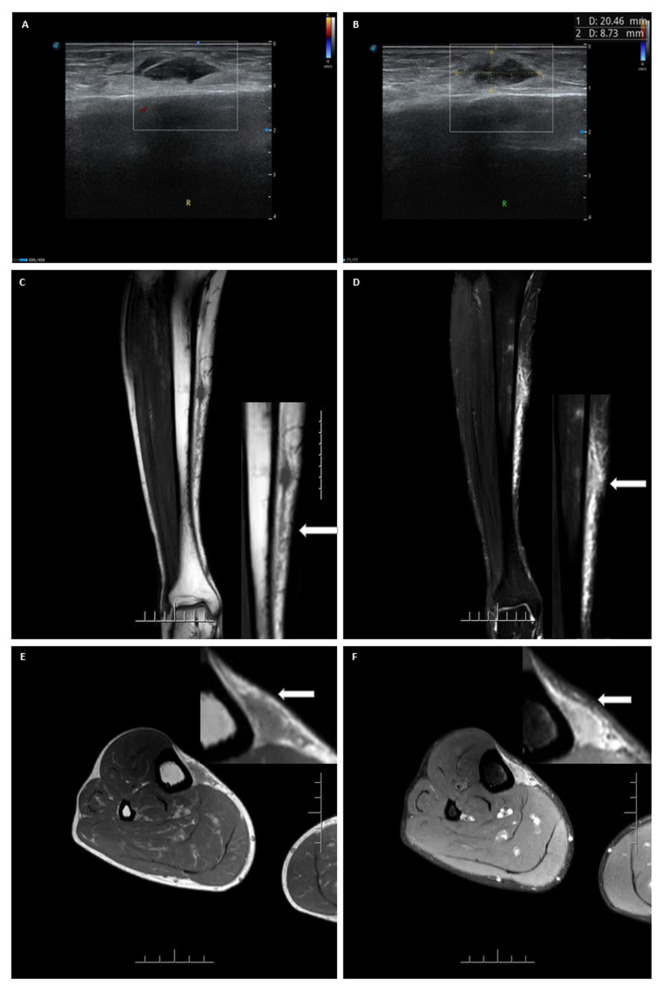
(A, B) Ultrasound (B-mode and CDFI) demonstrating a solid, well-circumscribed subcutaneous mass in the right anterior tibial region measuring 2.0 × 0.9 cm, with internal star-shaped vascularity on color Doppler flow imaging. Magnetic resonance imaging of the right lower leg demonstrating the subcutaneous pretibial nodule. (C) T1-sagittal image: the nodule (arrowhead) measures approximately 1.6 × 0.9 cm in cross-section and demonstrates signal intensity mildly higher than that of adjacent skeletal muscle, with relatively defined margins on this sequence. (D) T2 fat-suppressed sagittal image: the nodule (arrowhead) shows markedly increased signal intensity with ill-defined peripheral margins and adjacent soft tissue edema, consistent with peritumoral inflammatory infiltration. (E) T2-weighted sequence, axial plane. (F) T2 fat-suppressed axial image: the longitudinal extent of the nodule (arrowhead, 0.8 cm in the craniocaudal dimension) is depicted with surrounding soft tissue signal abnormality. The lesion is located in the subcutaneous compartment superficial to the deep fascia, without involvement of the underlying periosteum, tibial cortex, or medullary cavity.
